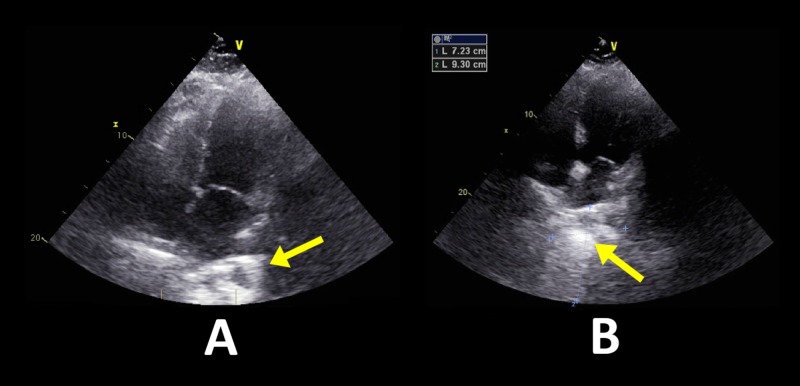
Transthoracic echocardiogram Left ventricular ejection fraction 65% with grade 1 diastolic dysfunction. A: Four chamber view at the end of diastole exhibiting no signs of encroachment upon the left atrium; B: Four chamber view at the end of systole better visualizing an echogenic mass just anterior to the left atrium.
